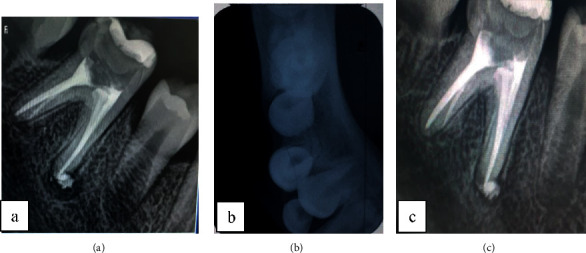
(a) Reduction of the periapical lesion size on the periapical radiograph after 8 months. (b) Occlusal radiograph showed total resolution of the periostitis after 8 months. (c) Periapical radiograph after 12 months: total regression of the apical radiolucency.
